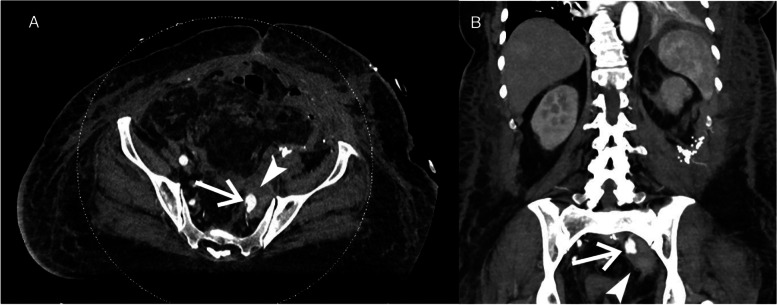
Initial CT angiogram of the left internal iliac artery mycotic pseudoaneurysm. A) Axial CT image and B) MPR coronal CT image shows left internal iliac artery mycotic pseudoaneurysm measuring 3 × 6 cm(arrow) and adjacent inflammatory changes (arrowhead)
